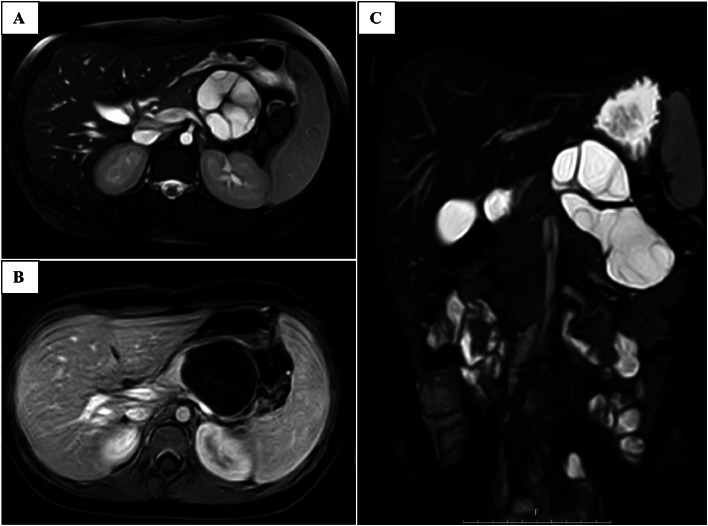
(A) Axial plane of fat saturated T2W MRI, (B) Axial plane of fat saturated T1W MRI with contrast, (C) Coronal plane of fat saturated T2 MRI. Images showed a multiloculated cystic lesion with benign appearance without solid component in the body and tail of pancreas. The lesion showed no enhancing septa or wall.
